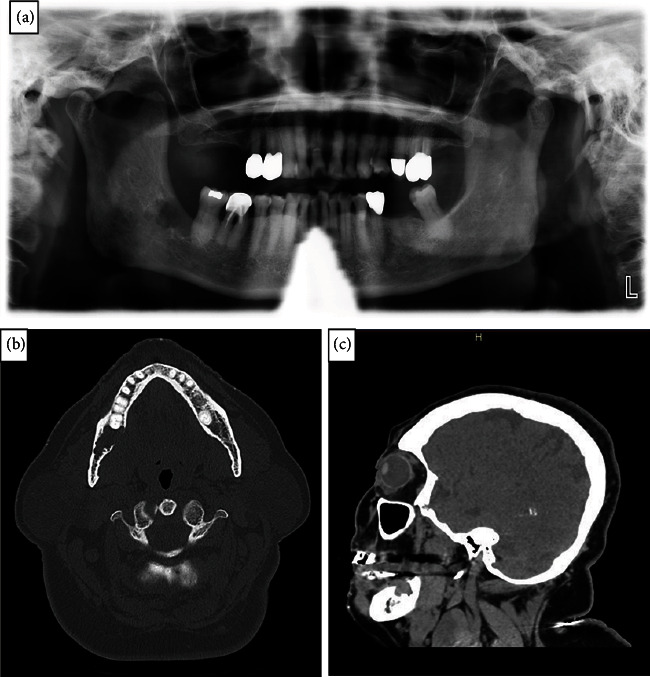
Radiologic images of a *MAML2-*rearranged central mucoepidermoid carcinoma. (a) Panoramic radiograph showing an ill-defined unilocular radiolucency distal to tooth #31. (b) Axial view of a CT-scan shows a unilocular radiolucency distal to tooth #31 with erosion of the lingual cortical plate and thinning of the buccal cortical plate. (c) Sagittal view of a CT-scan shows a unilocular radiolucency.
